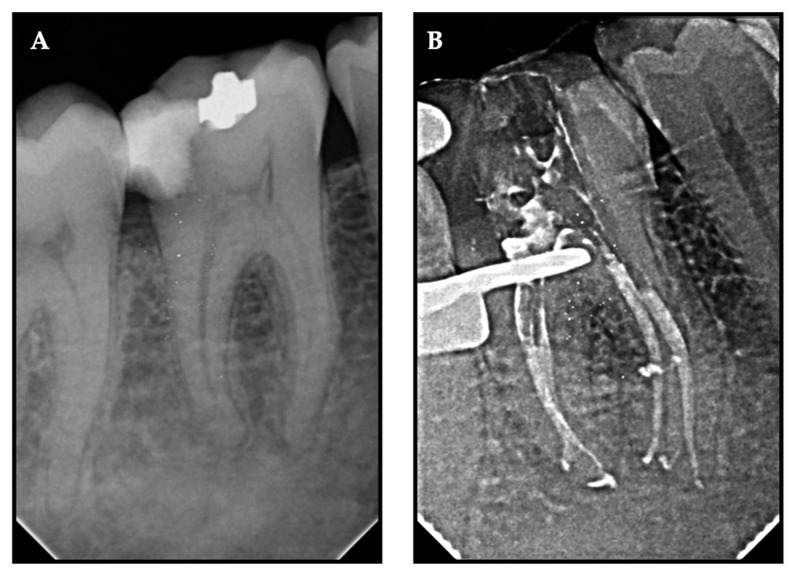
Representative radiovisiographs (RVGs) of (A) pre-operative and (B) post-obturation X-ray exhibiting sealer extrusion.
